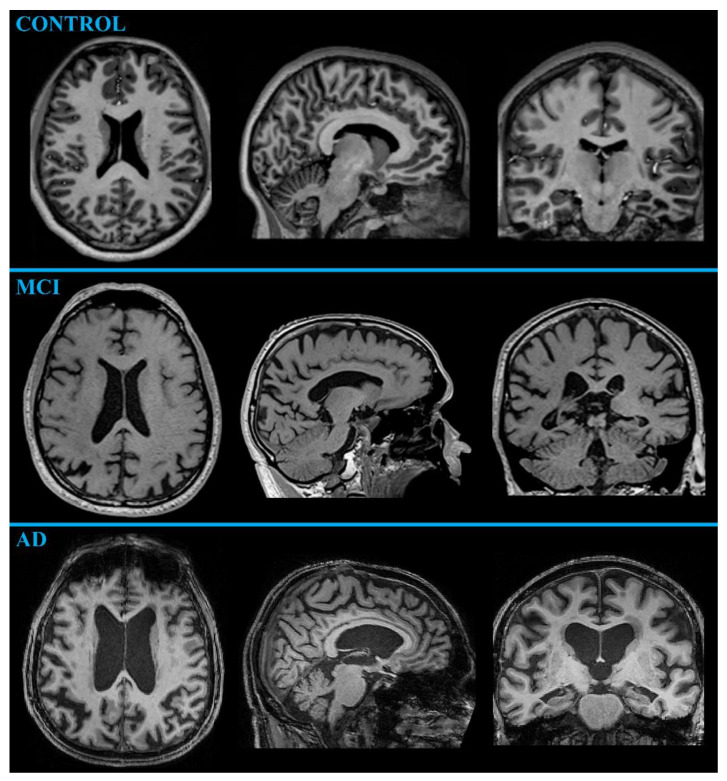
Example of MR neuroimaging (T_1_-weighted MRI at 3 Tesla MR-scanner) of a control subject (70-year-old female), of an MCI patient proceeding to AD in two years (72-year-old male), and of an AD patient (75-year-old female) in axial, sagittal, and coronal sections. The differences are visually subtle, but the increased atrophy in the medial temporal lobe and the enlarged ventricles are apparent in MRI.
